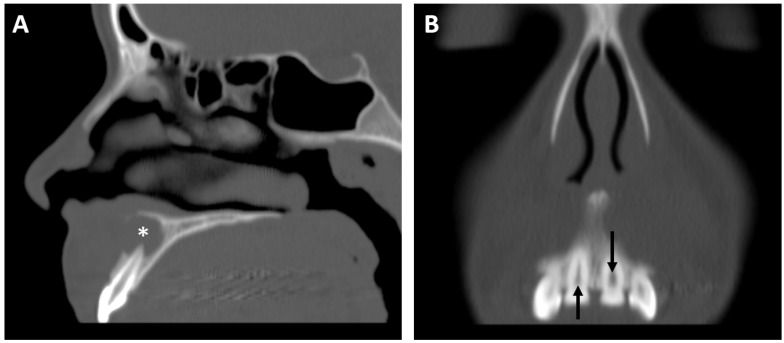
An 18-year-old female with tooth pain. (A) Sagittal-reformatted CT shown in bone windows reveals a periapical lucency (asterisk) involving the left maxillary central incisor, compatible with a cyst, granuloma, or abscess, in keeping with a diagnosis of periapical periodontitis. (B) Coronal-reformatted CT demonstrating a widened pulp chamber of the left maxillary central incisor (down arrow) relative to the right maxillary central incisor (up arrow), indicative of remote/chronic pulpal necrosis.
